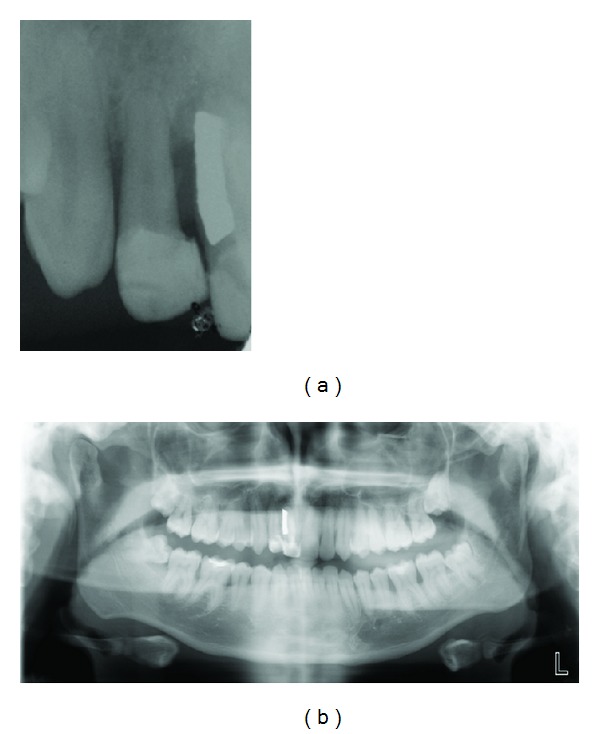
Periapical and panoramic radiographs showed a severe vertical bone defect.
